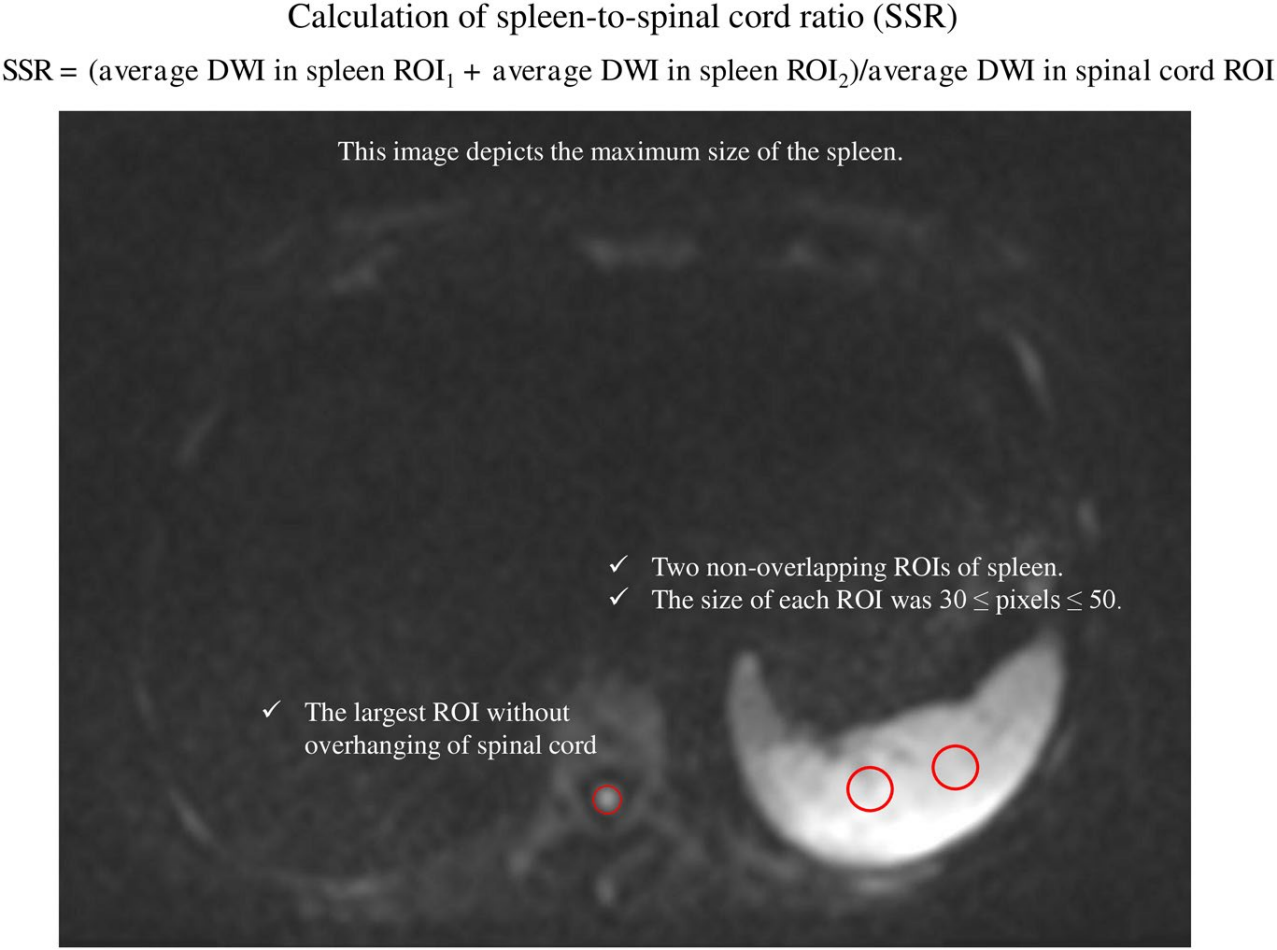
Calculation of spleen-to-spinal cord ratio. The spleen-to-spinal cord ratio (SSR) is calculated as the sum of the average diffusion-weighted imaging (DWI) in spleen (region of interest) ROI_1_ and spleen ROI_2_ divided by the average DWI in the spinal cord ROI.
